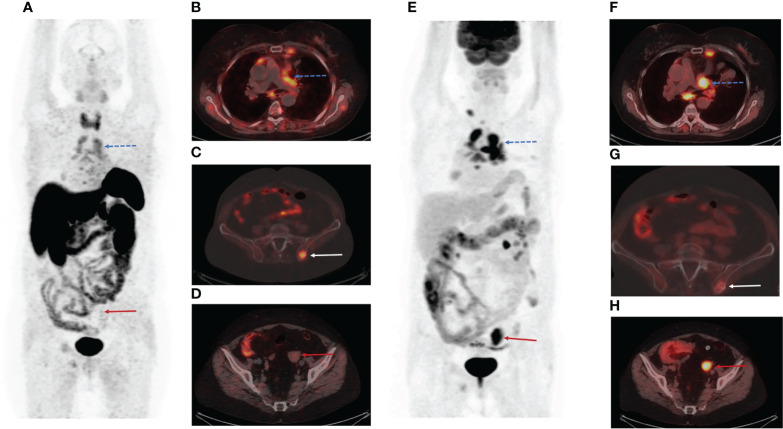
Positron emission tomography/computed tomography (PET/CT) images with Gallium-68 [68Ga]-labeled dodecanetetraacetic acid-tyrosine-3-octreotate ([68Ga]-DOTA-TATE) and Fluor-18 [18F]-fluorodeoxyglucose ([18F]-FDG). (A) The maximum intensity projection (MIP) images summarize the physiological and pathological distribution of [68Ga]-DOTA-TATE in the body. (B) PET/CT scan showed multiple mediastinal lymphatic nodes (blue dotted arrow) and widespread bone metastases with significant uptake of [68Ga]-DOTA-TATE. (C) [68Ga]-DOTA-TATE-positive bone metastasis in the left iliac wing (white arrow). (D) [68Ga]-DOTA-TATE-negative solid mass in the left ovary (red arrow). (E) The MIP images summarize the physiological and pathological distribution of [18F]-FDG in the body. (F) Multiple [68Ga]-DOTA-TATE-positive metastases in mediastinal lymphatic nodes (blue dotted arrow). (G) The axial PET/CT image demonstrated low [18F]-FDG uptake in the bone metastasis in the left iliac wing (white arrow). (H) The axial slice of [18F]-FDG PET/CT showed high [18F]-FDG uptake in the solid mass in the left ovary (red arrow).
